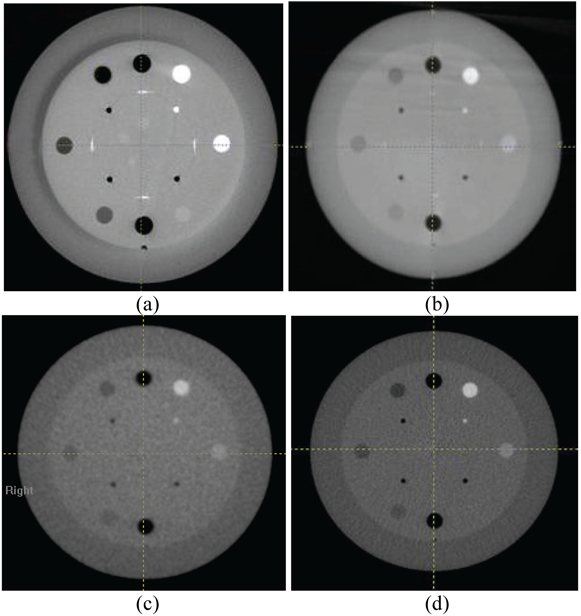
Images of CTP404 module of the Catphan acquired to determine contrast linearity with the four CBCT/FBCT systems; a) Varian kV CBCT; b) Elekta kV CBCT; c) Siemens MV CBCT; d) Tomo MV FBCT.
